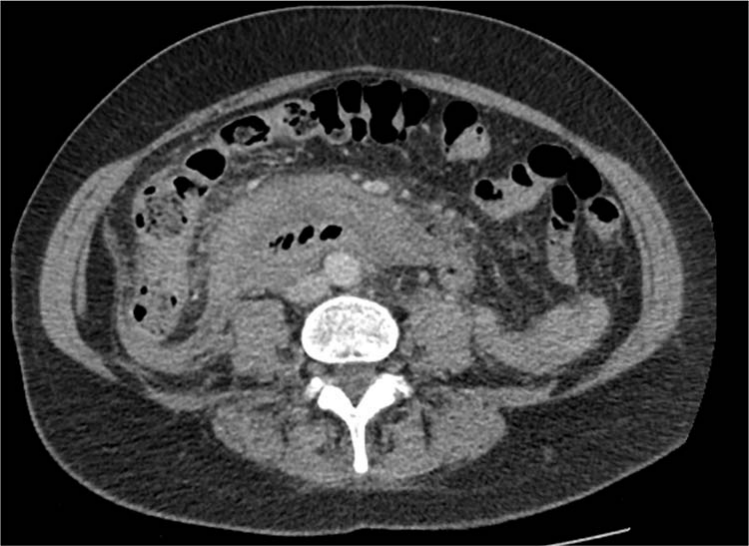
CT abdomen, axial view. Hyperdense fluid centered around the duodenum and tracking into the paracolic gutters. Appearance concerning for paraduodenal hemorrhage, infiltrating mass was considered less likely.
